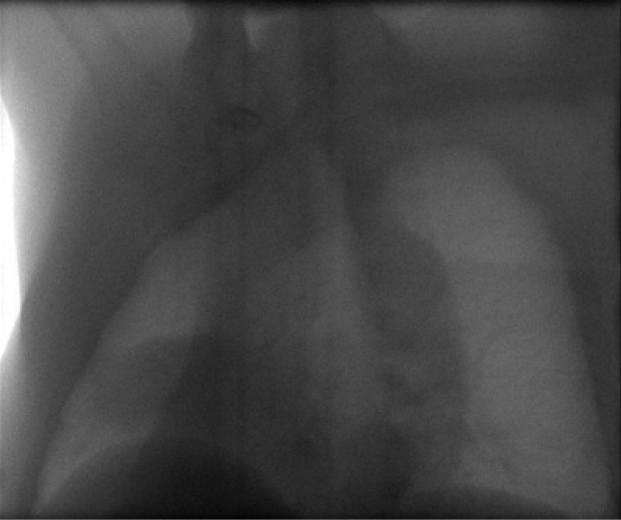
Projection image from the MVCBCT raw dataset of a NSCLC patient. The tumor is visible in the right lung just above the diaphragm.
